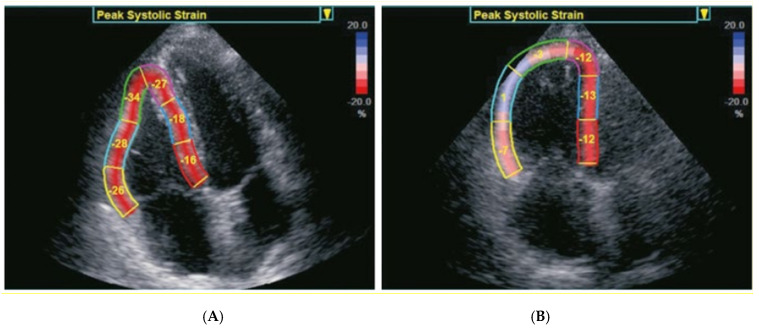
Examples of right ventricular strain analysis by speckle-tracking echocardiography. (A) Patient with a normal RV function. (B) Patient with a reduced RV function.
